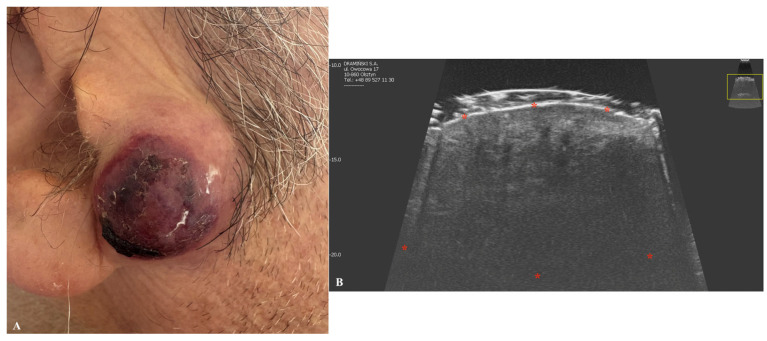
Macroscopic view of dermatofibrosarcoma protuberans (A) with a corresponding ultrasound image (B). Stars indicate tumor margins. The yellow box indicates the area covered by the ultrasonographic field of view.
